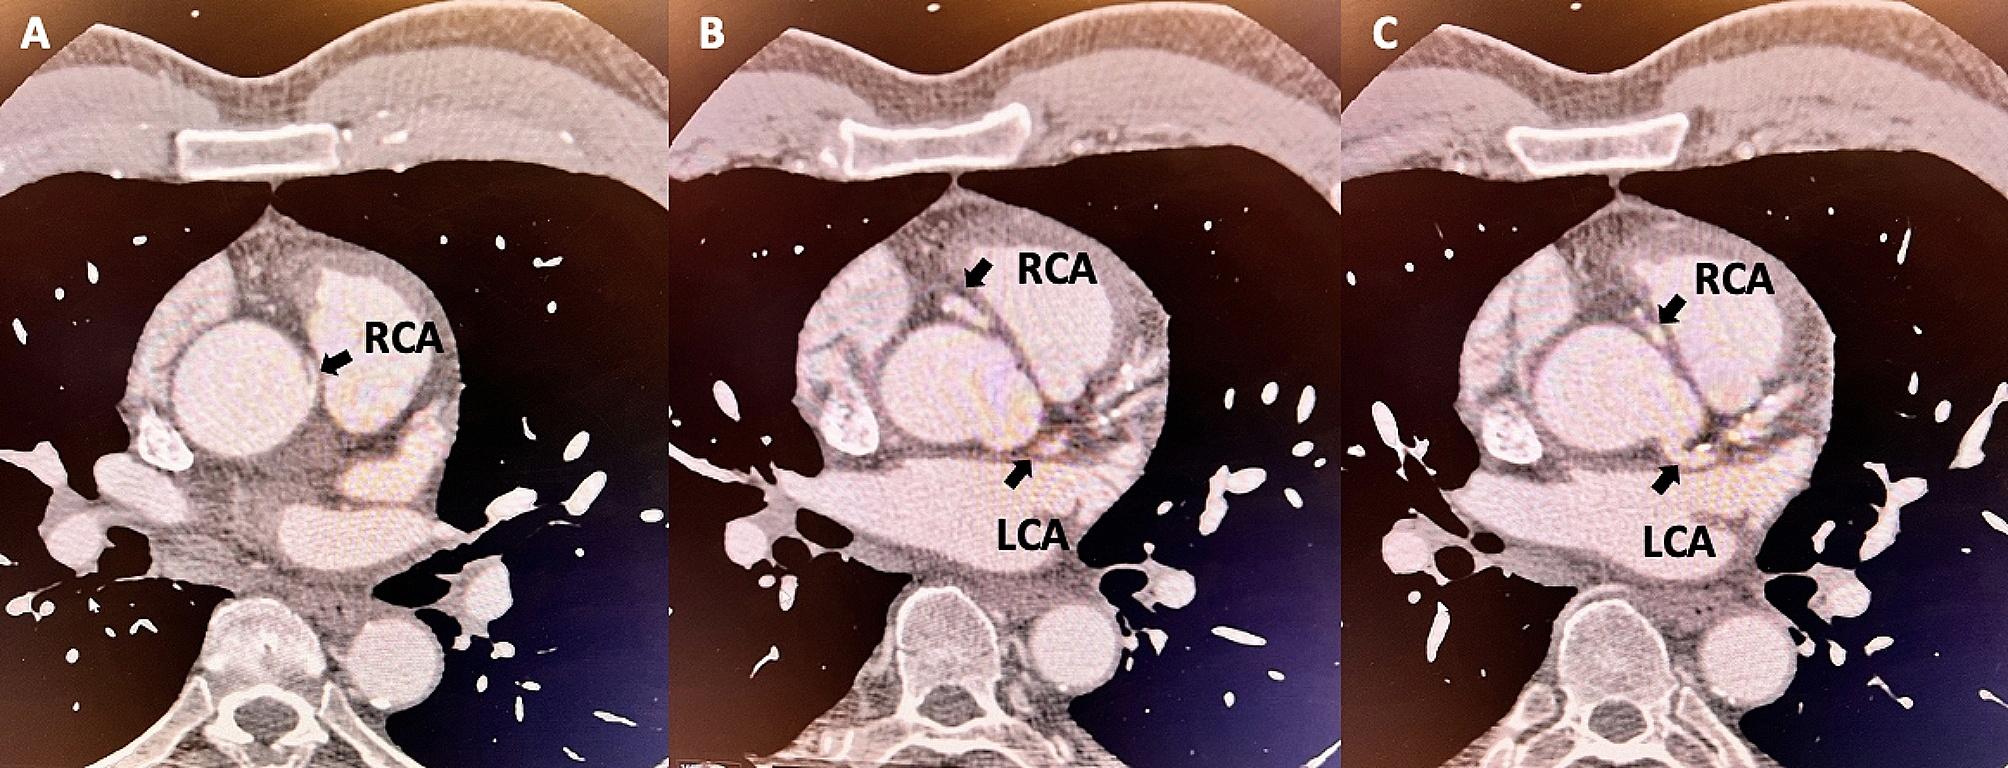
Computed Tomography Angiography of the chest showing the origin of the right coronary artery (RCA) (A), its inter-arterial course (B), and the origin of the left coronary artery (LCA) (C)
